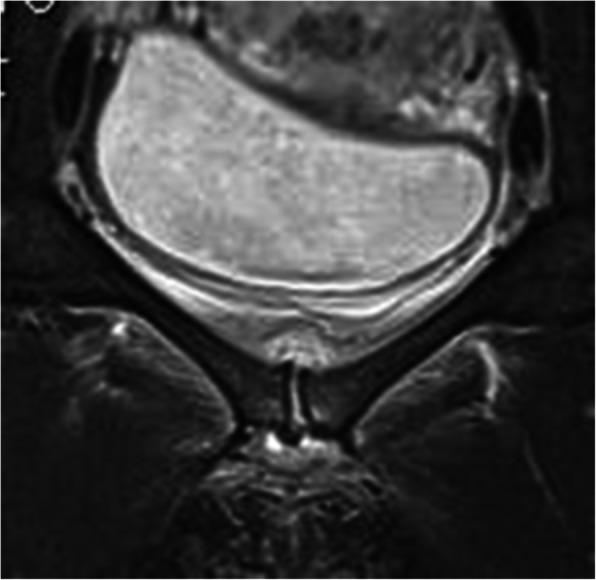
MRI showing oedema of the symphysis protruding both cranio-dorsally, approaching the urinary bladder, as well as caudally close to the urethra
